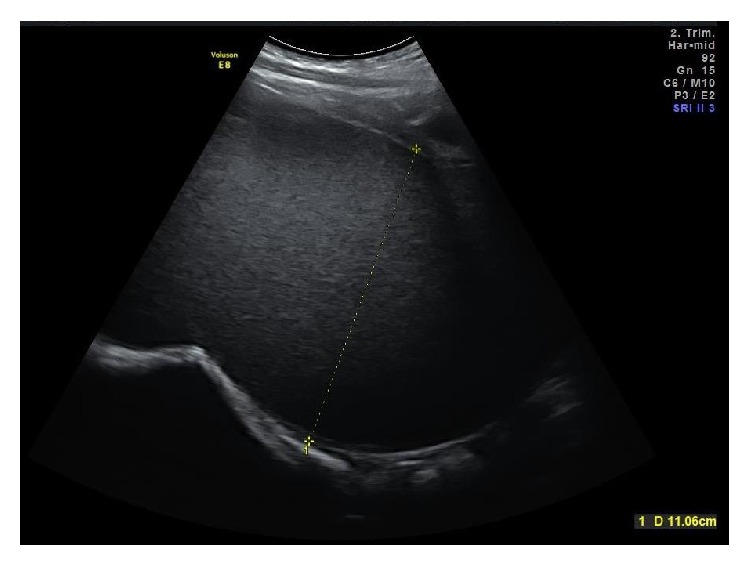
2D ultrasound imaging of megahematocolpos.
